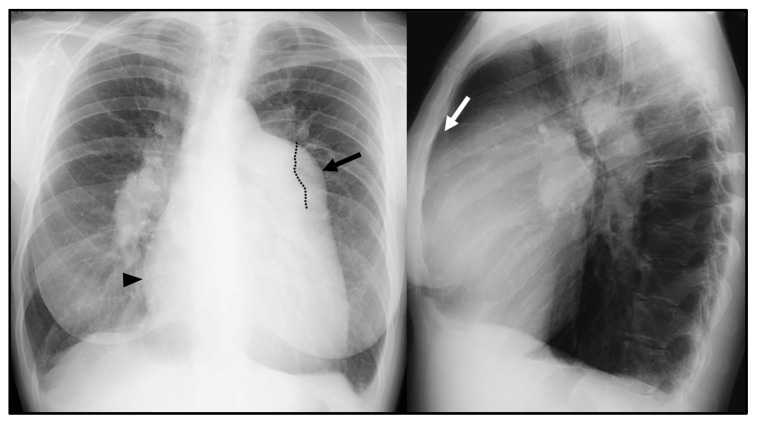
Chest X-ray (CXR) signs of pulmonary hypertension: enlarged central pulmonary arteries, with the prominence of the main pulmonary artery (MPA) (black arrow); the left pulmonary artery seen within the cardiac silhouette (dotted line); tapering of peripheral pulmonary arteries (so-called *pruning*); prominence of the right heart border, represented by the right atrium (black arrowhead); and filling of the retrosternal space in the lateral view due to right ventricle dilation (white arrow).
